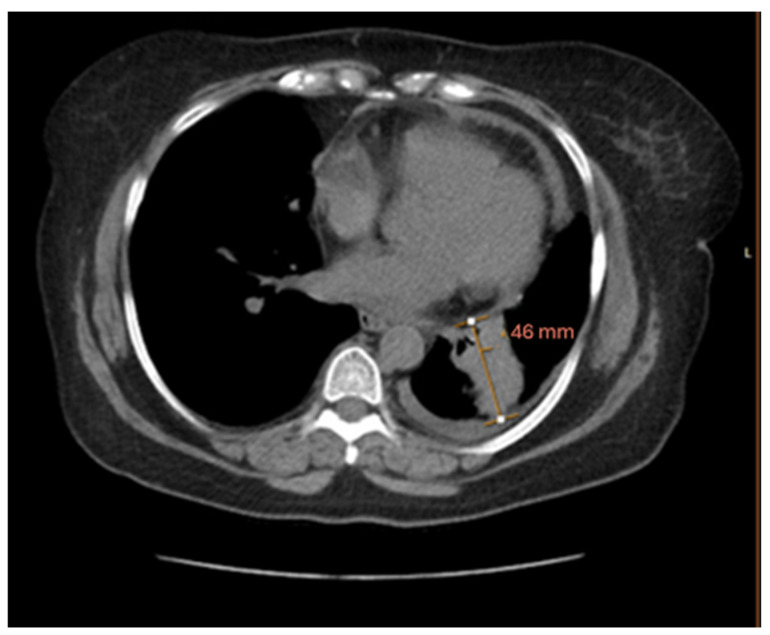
CT-scan at the time of diagnosis showing a left lower lobe perihilar soft tissue mass lesion.
